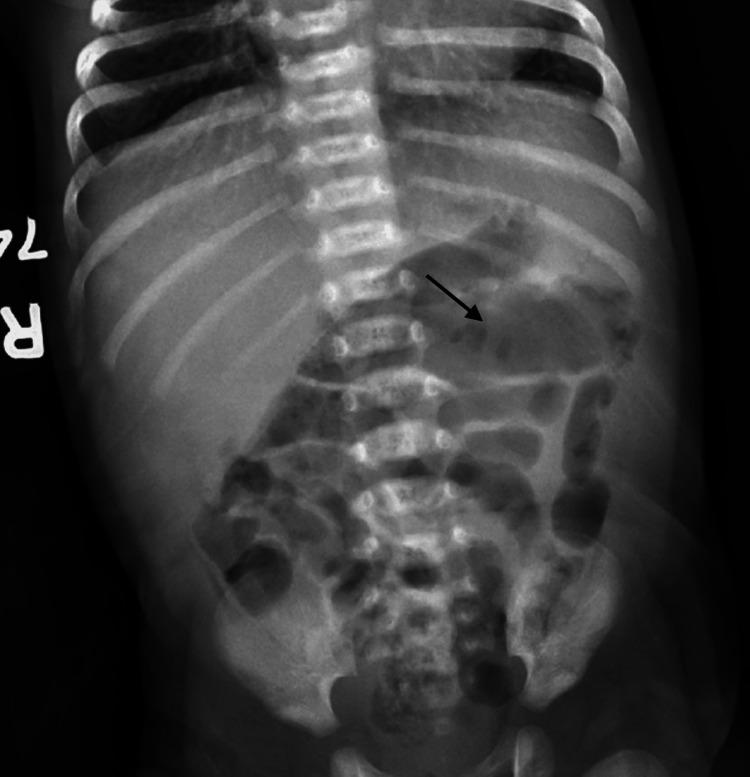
Initial X-ray of the Abdomen with arrow showing possible intramural air in the ascending colon raising concern for pneumatosis, finding that can be associated with necrotizing enterocolitis.
